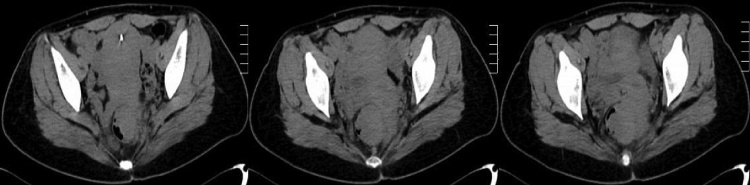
CT showed the rectal occupying lesions.
